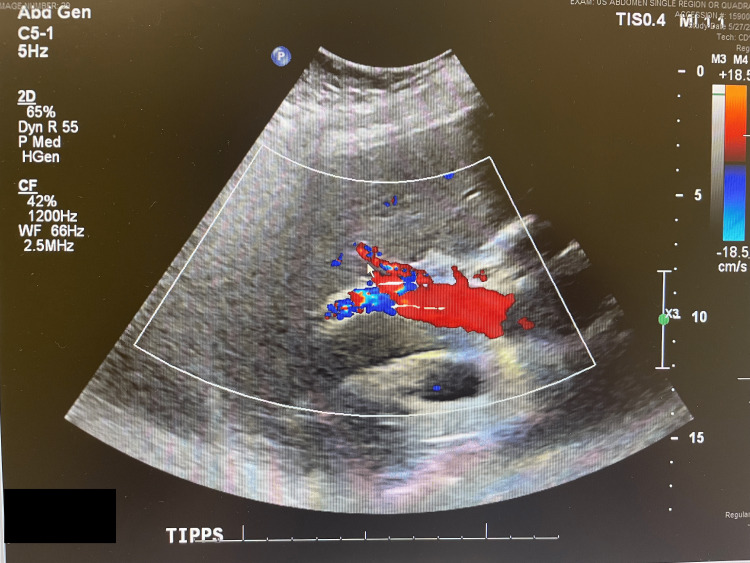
Patient's echocardiogram during her admission
